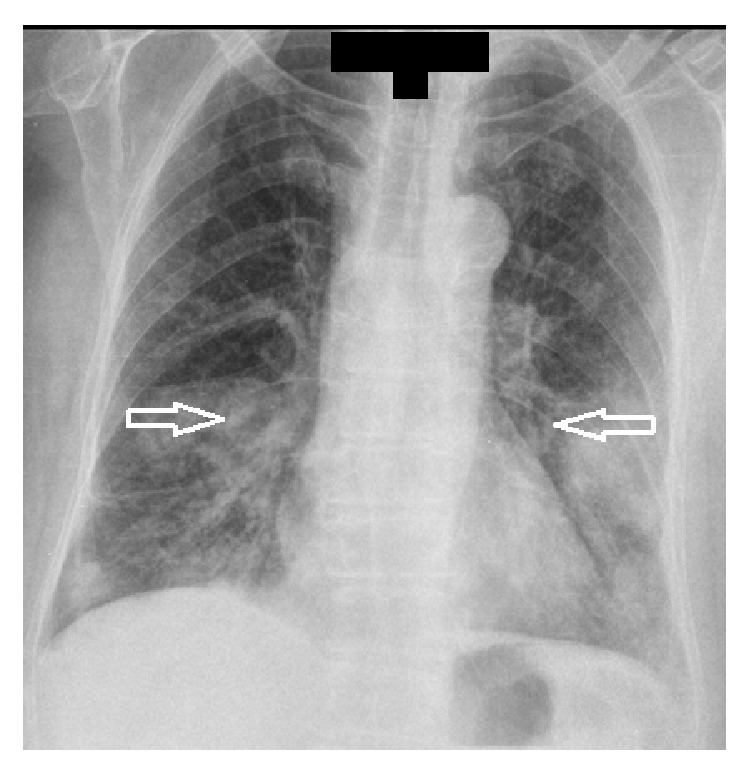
Posteroanterior chest X-ray showing parenchymal infiltrations and cavitary lesions.
